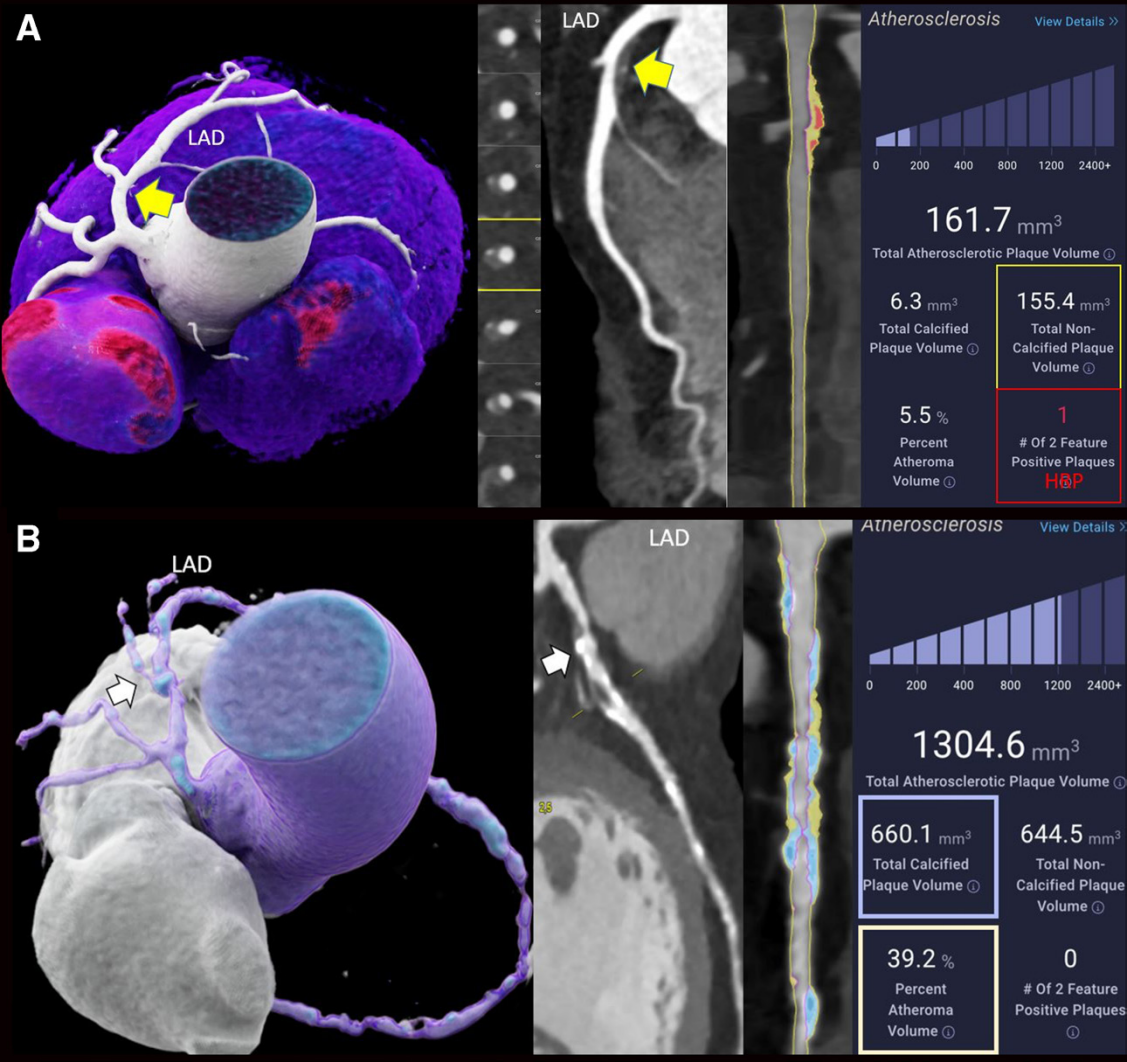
Case examples. A, A 55-year-old woman with atypical chest pain and 2 cardiovascular risk factors (CVRFs): arterial hypertension, dyslipidemia, obesity, and hyperuricemia. Coronary computed tomography angiography (CTA) showed a noncalcified plaque (NCP) in the proximal left anterior descending (LAD) coronary artery (yellow arrow) with <50% diameter stenosis. Treatment with lipid-lowering therapy (statin+ezetimibe) and antihypertensive medication was initiated, and she remained event-free. Right, Artificial intelligence–based quantitative computed tomography (AI-QCT) plaque analysis showed an NCP volume of 155.4 mm^3^ (yellow box) and high-risk-plaque (HRP; red box) features. Note that color overlay (mid) facilitates the delineation of NCP and HRP. B, A 65-year-old woman with atypical chest pain, dyspnea, and a high coronary risk profile (5 CVRFs), smoking, arterial hypertension, positive family history, dyslipidemia, diabetes, and high lipoprotein A (89.1 ng/dL), who experienced major adverse cardiovascular event and underwent percutaneous coronary intervention (PCI) (LAD prox/mid/distal and right coronary artery [RCA]). Coronary CTA: white arrow pointing at calcified plaque in the proximal LAD. Mid, Curved multiplanar reformation. Right, AI-QCT plaque analysis revealed high total plaque volume (stage 3) with high calcified, high noncalcified plaque volume, and high percentage atheroma volume (39.2%).
